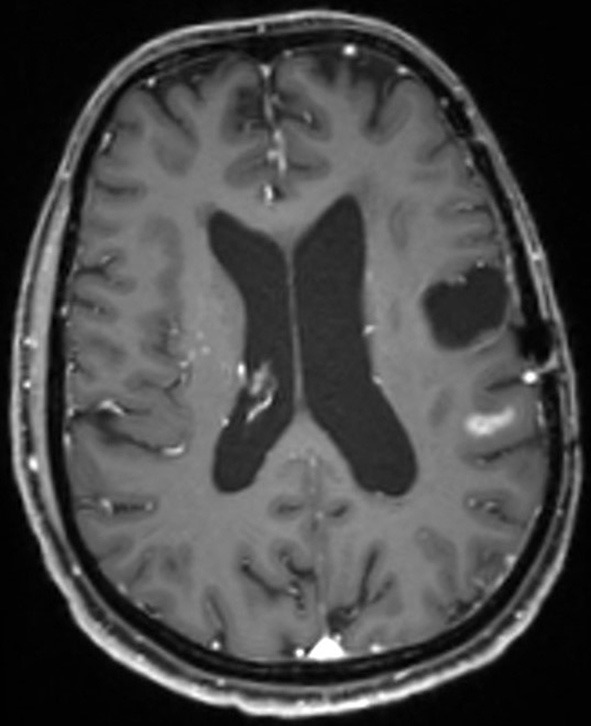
Pre-operative MR image (T1-weighted sequence, axial plane) showing new contrast-enhancement distant to the former resection cavity.
